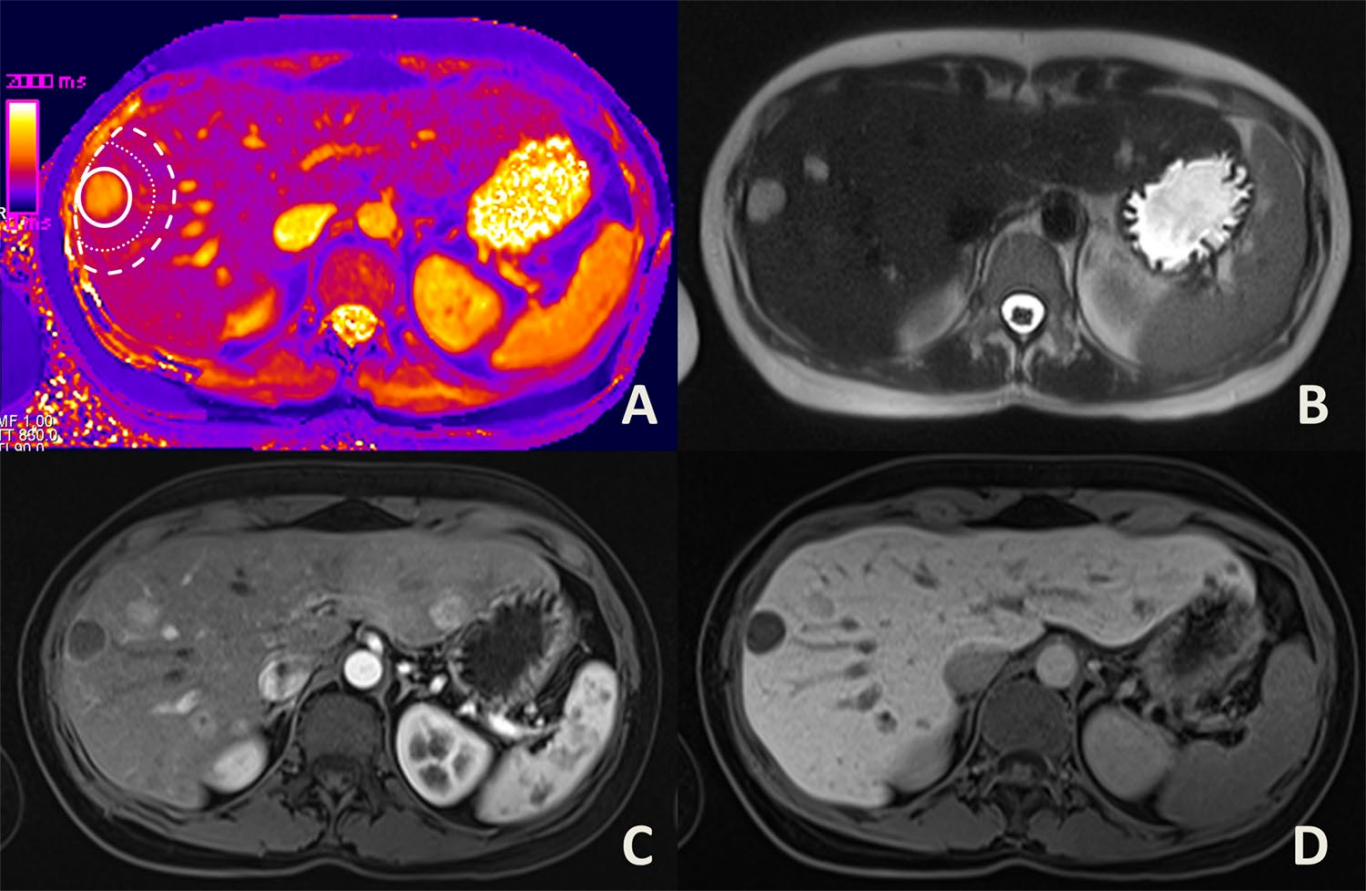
Placement of a region of interest (A) around a metastasis in a 33 year-old female patient with squamous cell carcinoma of the nasal sinus. As the lesion is located subcapsular, the peripheral zones (dotted and dashed circles) are placed excentrically around the lesion ROI (continuous circle), under careful avoidance of larger vessels, other focal lesions or extrahepatic structures. Additionally, a T2-weighted (B), an arterial phase (C), and the hepatobiliary phase (D) images are given.
